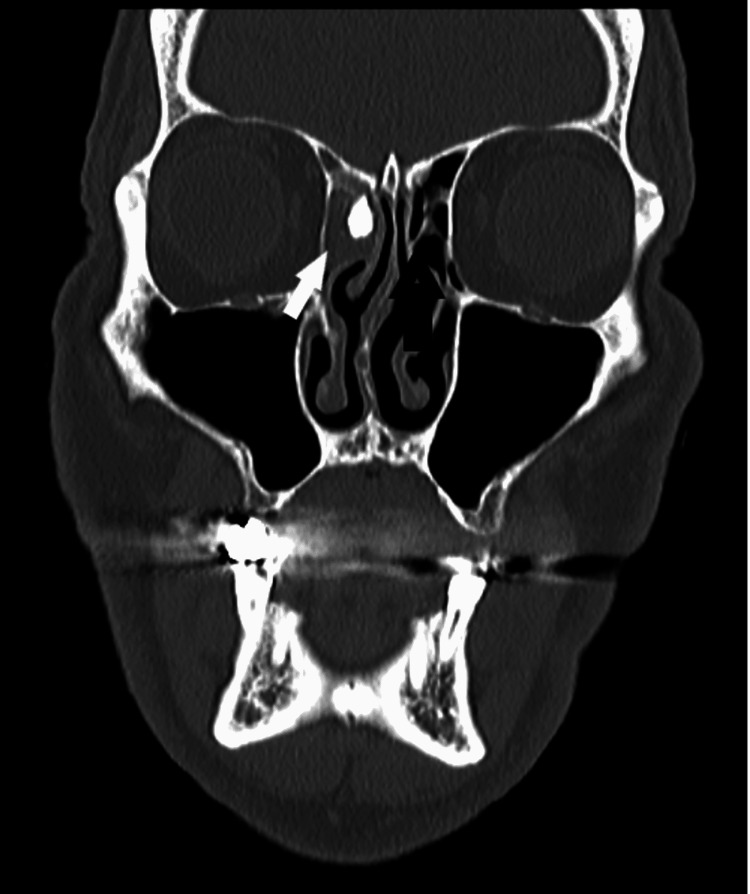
Bone window of the coronal computed tomography (CT) scan revealed a hyperdense calcified structure (arrow), consistent with a retained foreign body at the upper part of the anterior ethmoid cells.
